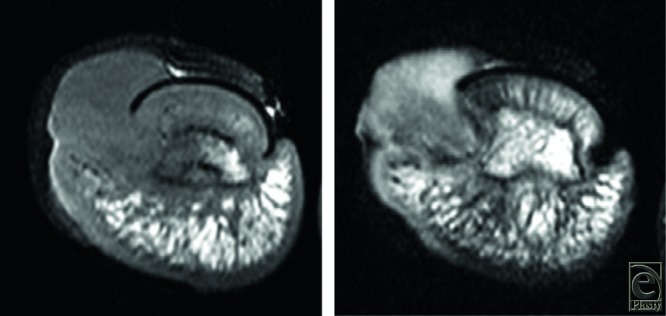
Magnetic resonance imaging revealed a mass occupying the medial aspect of the distal phalanx, with low signal intensity on T1-weighted images and intermediate to high signal intensity on T2-weighted images.
